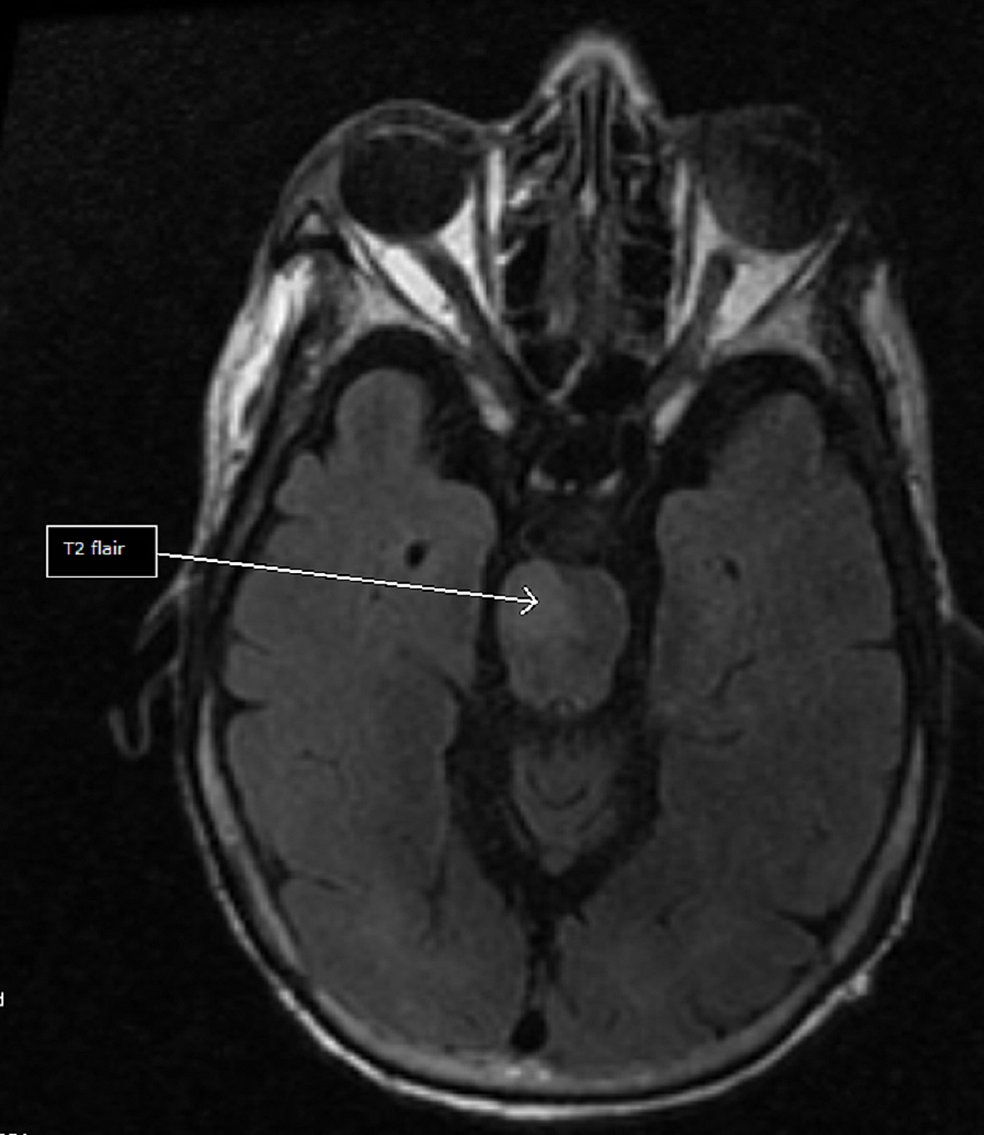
T2 FLAIR MRI imaging. FLAIR: Fluid attenuated inversion recovery.
